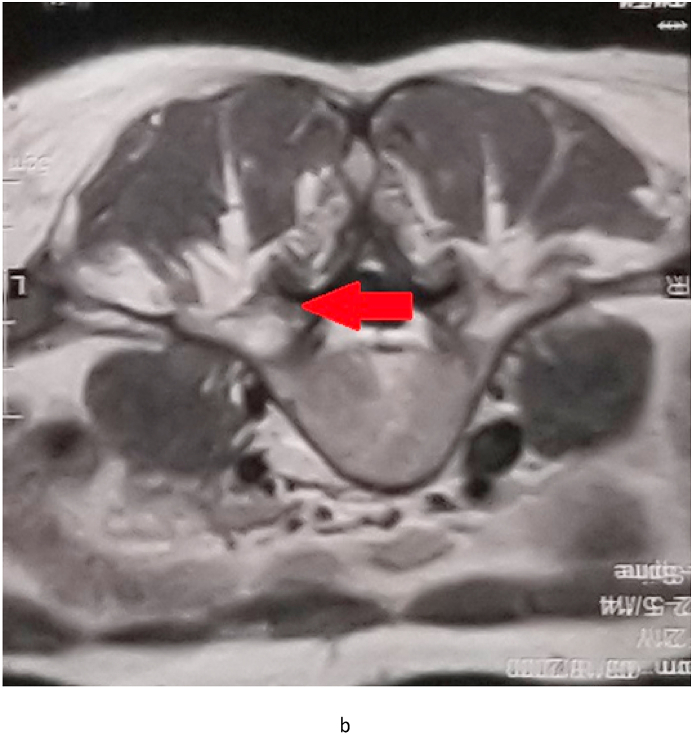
In Axial MRI, the red arrow shows a lesion on the posterior side of the vertebra with severe central canal stenosis. (For interpretation of the references to colour in this figure legend, the reader is referred to the Web version of this article.)
